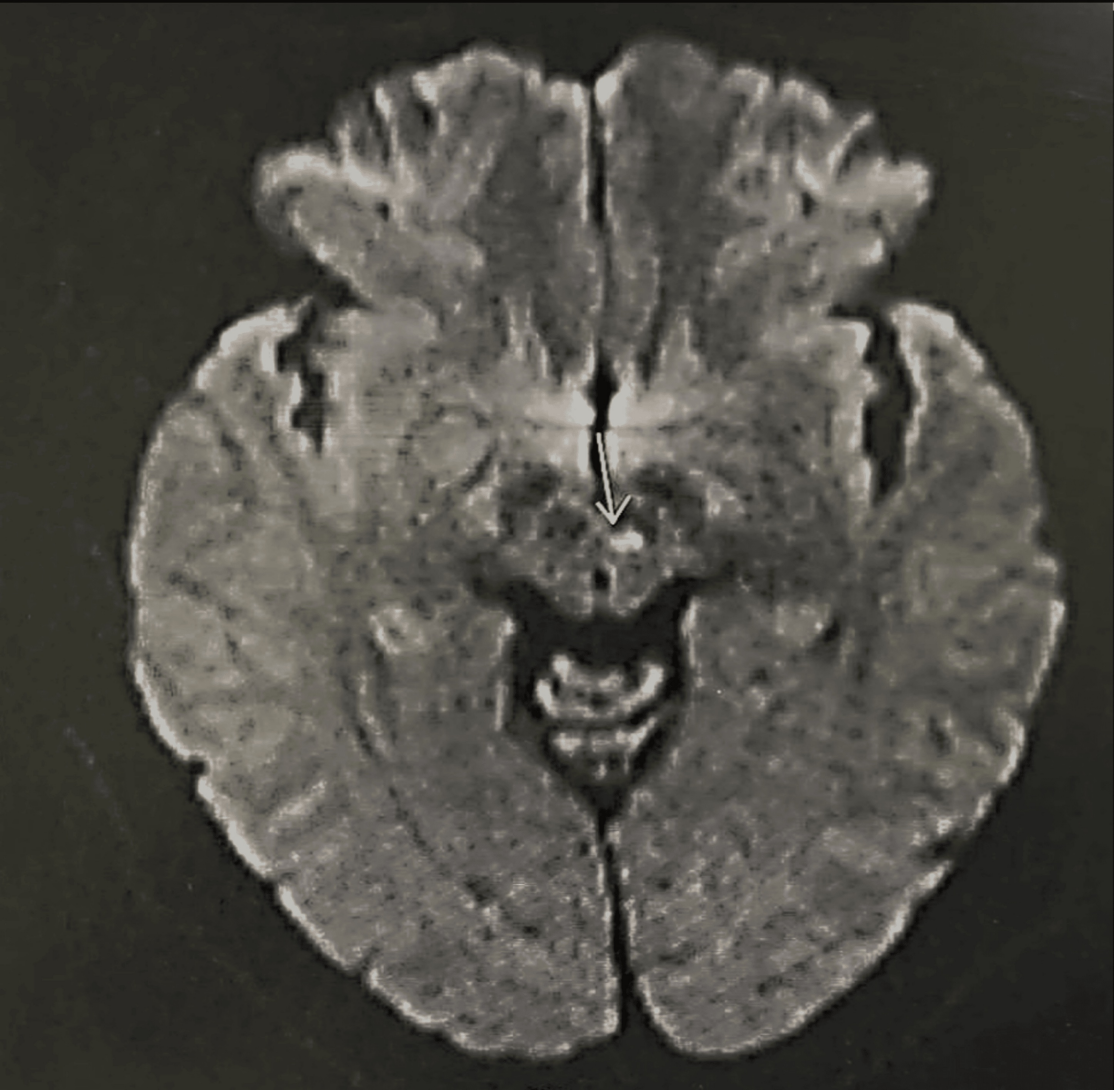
Magnetic resonance imaging shows recent midbrain lacunar infarcts.
